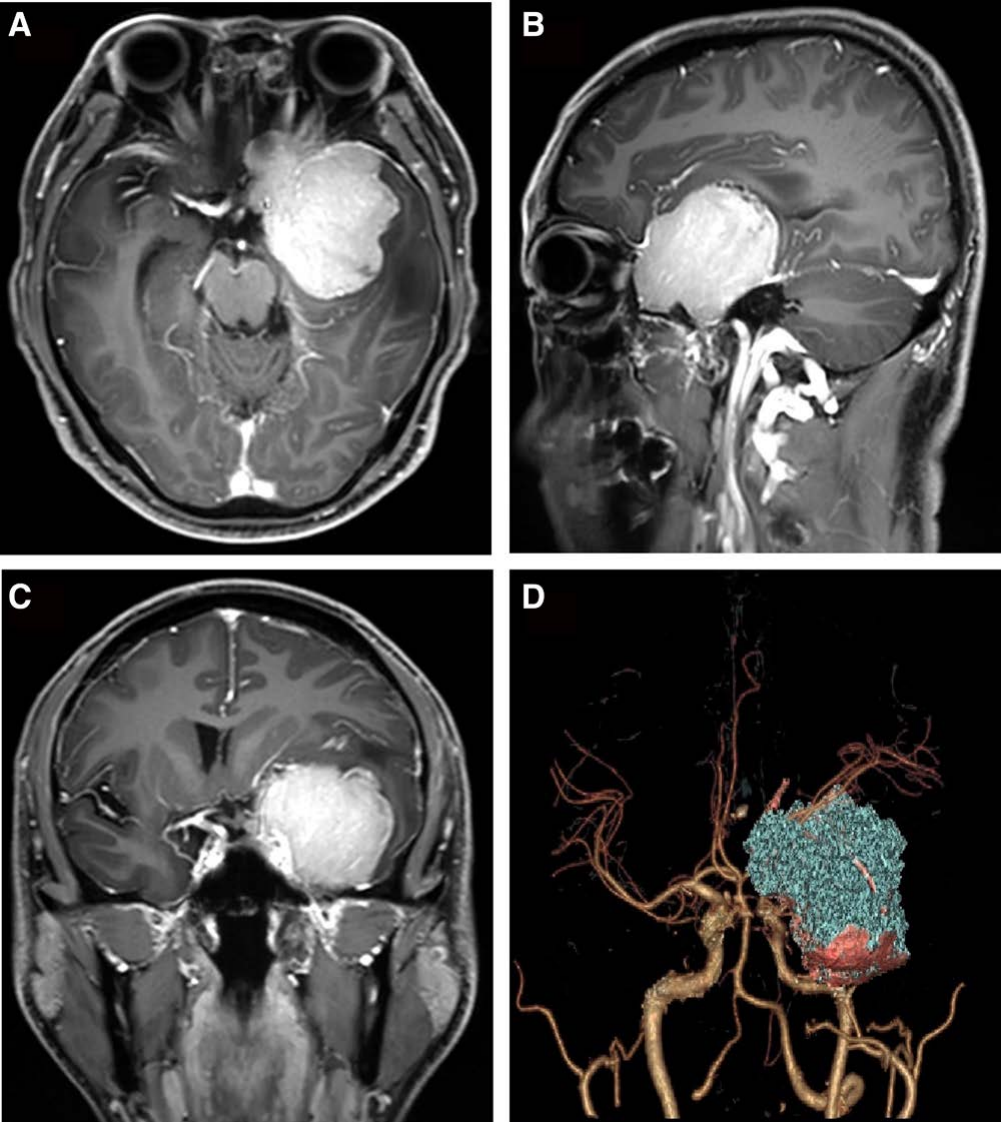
A preoperative MRI of the head showed a space-occupying lesion in the left middle cranial base or the sphenoid ridge. (A) Axial, (B) sagittal, (C) coronary. MRI = magnetic resonance imaging.
